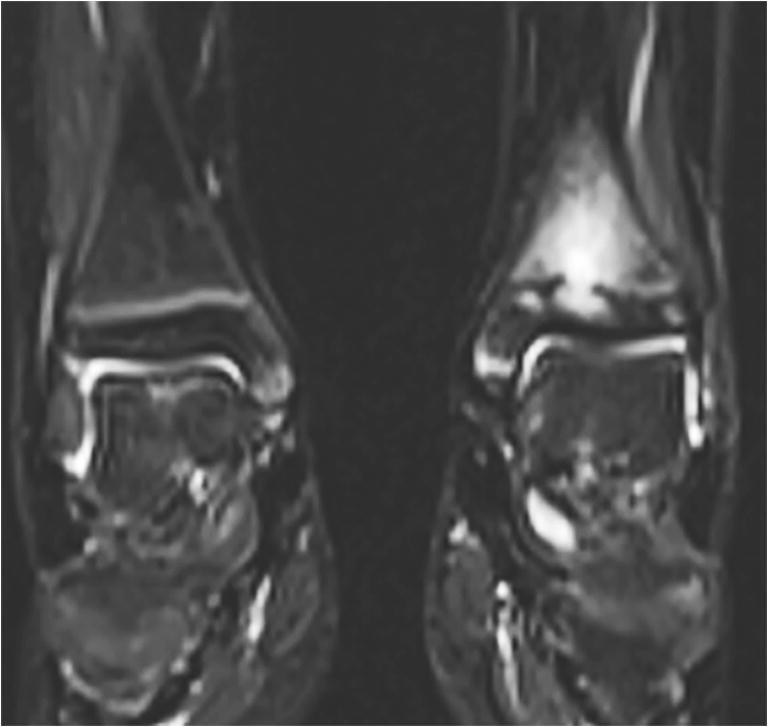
Chronic nonbacterial osteomyelitis in a 9-year-old girl. Coronal T2-W short tau inversion recovery MR image (repetition time/echo time = 5,000/58 ms) of both ankles shows involvement of the left metaphysis, growth plate and epiphysis. The right side is normal
